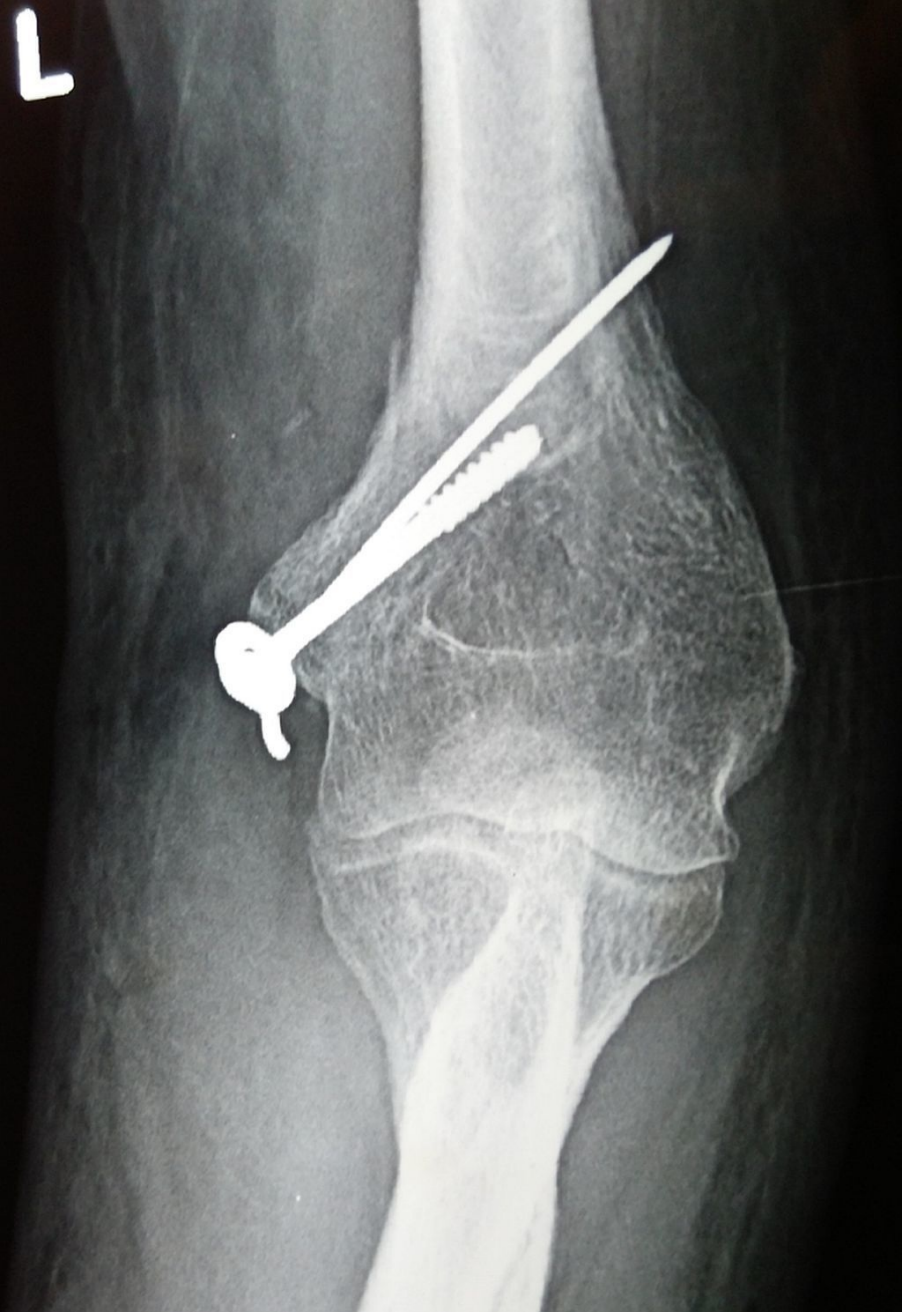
Anteroposterior radiograph of the elbow showing a reduced elbow joint with the screw and K-wire in situ.
